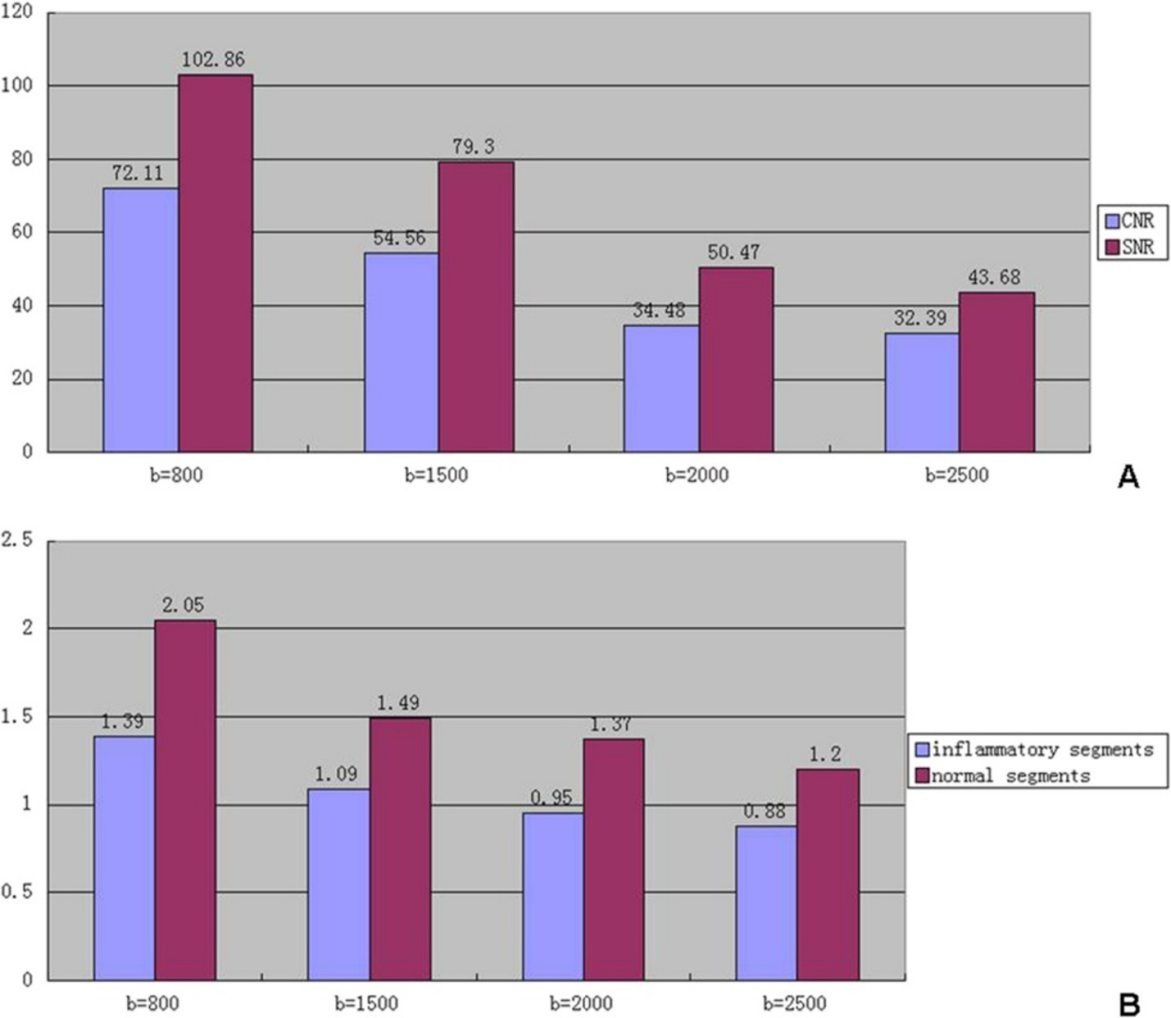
Determine the optimal b value in DWI with a learning cohort of patients. The mean CNR and SNR of four b value sequences. The unit of b value is s/mm^2^(A). The ADC values of four b value sequences. The unit of ADC is 10^−3^ mm^2^/s (B).
